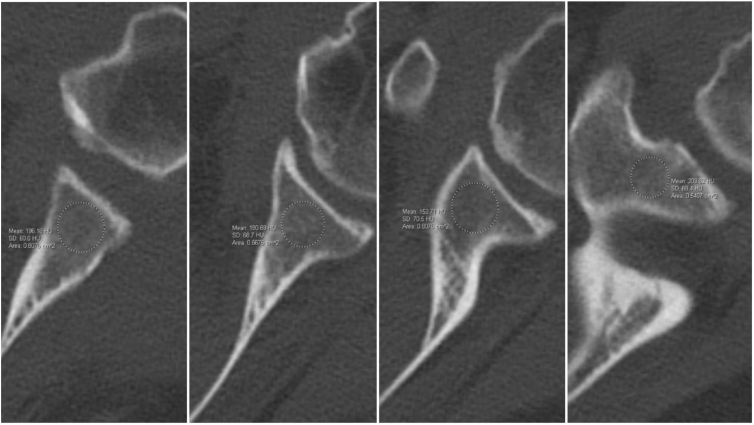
Measurement of HU from caudal to cranial (left to right) on computed tomography. *HU*, Hounsfield unit.
